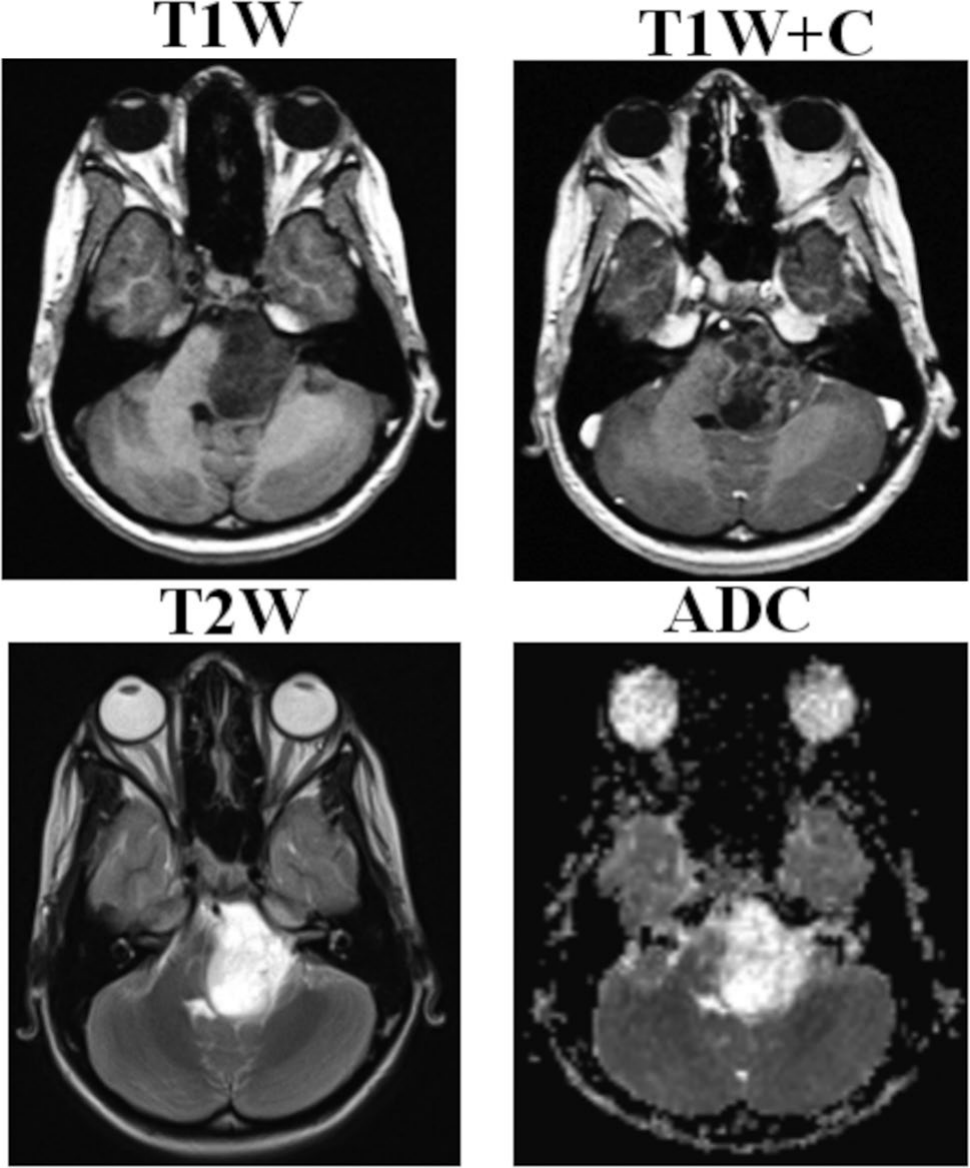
Pre-operative MRI imaging. Selected pre-operative MRI images demonstrating the exophytic tumour arising from the left pons. Note the presence of both solid enhancing components and non-enhancing cystic regions on the post-contrast T1-weighted imaging (T1W + C). Whilst there was some effacement of the IV^th^ ventricle by the tumour, there was no significant pre-operative hydrocephalus observed, and there were no other intracranial or spinal lesions detected. *ADC*, apparent diffusion coefficient; *T1W*, T1-weighted; *T1W* + *C*, post-contrast T1-weighted imaging; *T2W*, T2-weighted
